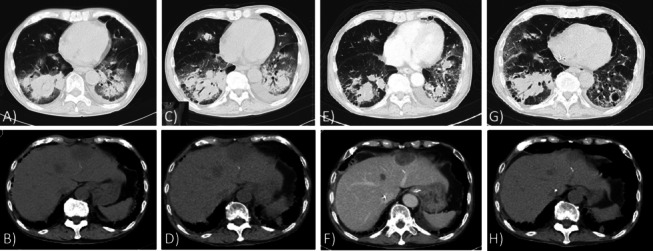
Radiological findings over time. A, B CT on admission revealed bilateral ground opacities superimposed with a subpleural reticular shadow and honeycombing. Multiple nodular lesions were detected in the bilateral lung fields and liver. C, D Re-examination of CT before intubation on the 4th hospital day showing the deterioration of opacities along with traction bronchiectasis. Pulmonary and liver nodules slightly increased in size. E, F On the 12th hospital day after weaning from the mechanical ventilator, diffuse ground-glass opacities improved and pulmonary and liver nodules decreased in size. G, H On the 21st hospital day before re-intubation, the interstitial shadow was exacerbated and the sizes of pulmonary nodules increased. Liver nodules showed no significant changes in size. Mediastinal emphysema was detected
